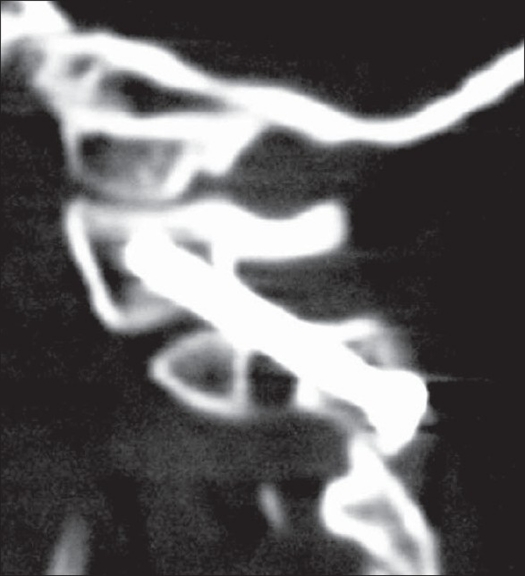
Postoperative CT scan of the patient shown in figure 3 with reconstruction showing the well positioned screws on either side
